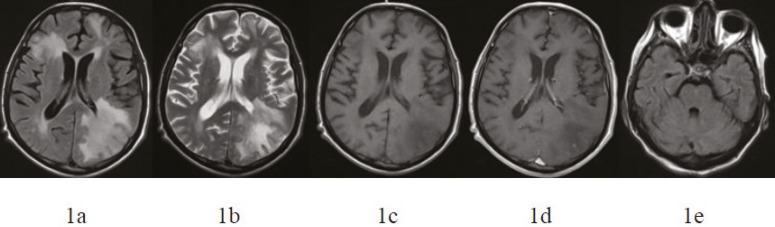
Axial fluid attenuated inversion recovery (FLAIR) (a) and axial T2-weighted images (b) show extensive hyperintense and axial T1-weighted image (c) shows hypointense lesions in the subcortical and periventricular white matter bilaterally. There is also compression of the posterior horn of the lateral ventricle due to mass effect. There is no remarkable contrast enhancement on T1-weighted postcontrast images (d). Axial FLAIR image (e) reveals that there is no lesion in the pons
